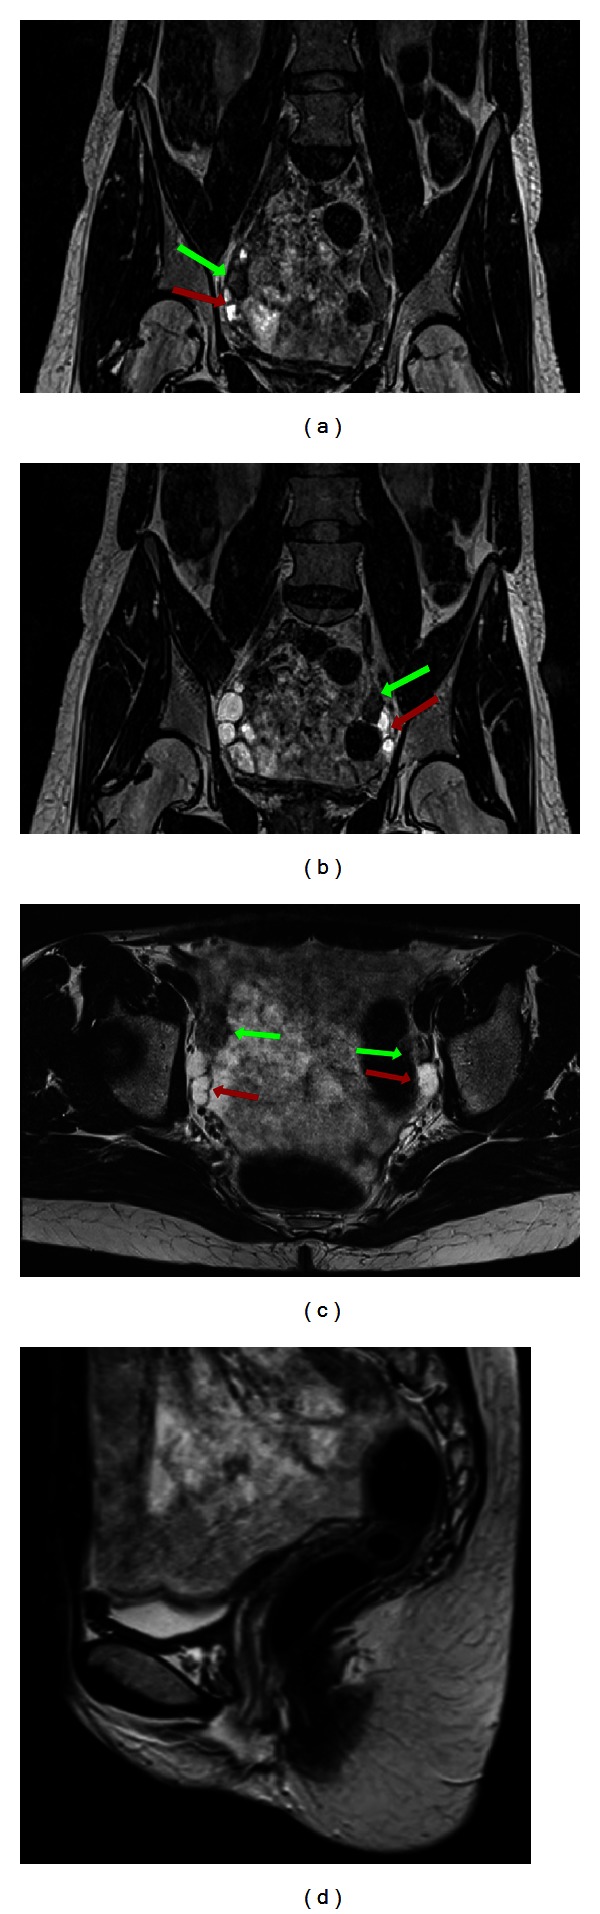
38-year-old girl/female affected by CAIS. MRI T2-weighted coronal ((a) and (b)) and axial (c) images demonstrating the presence of the testes (green arrows) and adjoining cysts (red arrows). Sagittal T2 weighted image (d) showing agenesis of the Müllerian structures and presence of the lower third of the vagina (yellow arrowheads). (3T Magnetic Resonance Imaging. Coronal 1 mm SPACE-sequence, TR/TE 1500/133; axial 4 mm fast-T2-weighted image, TR/TE 4500/119; sagittal 5 mm HASTE-sequence, TR/TE 6500/89).
